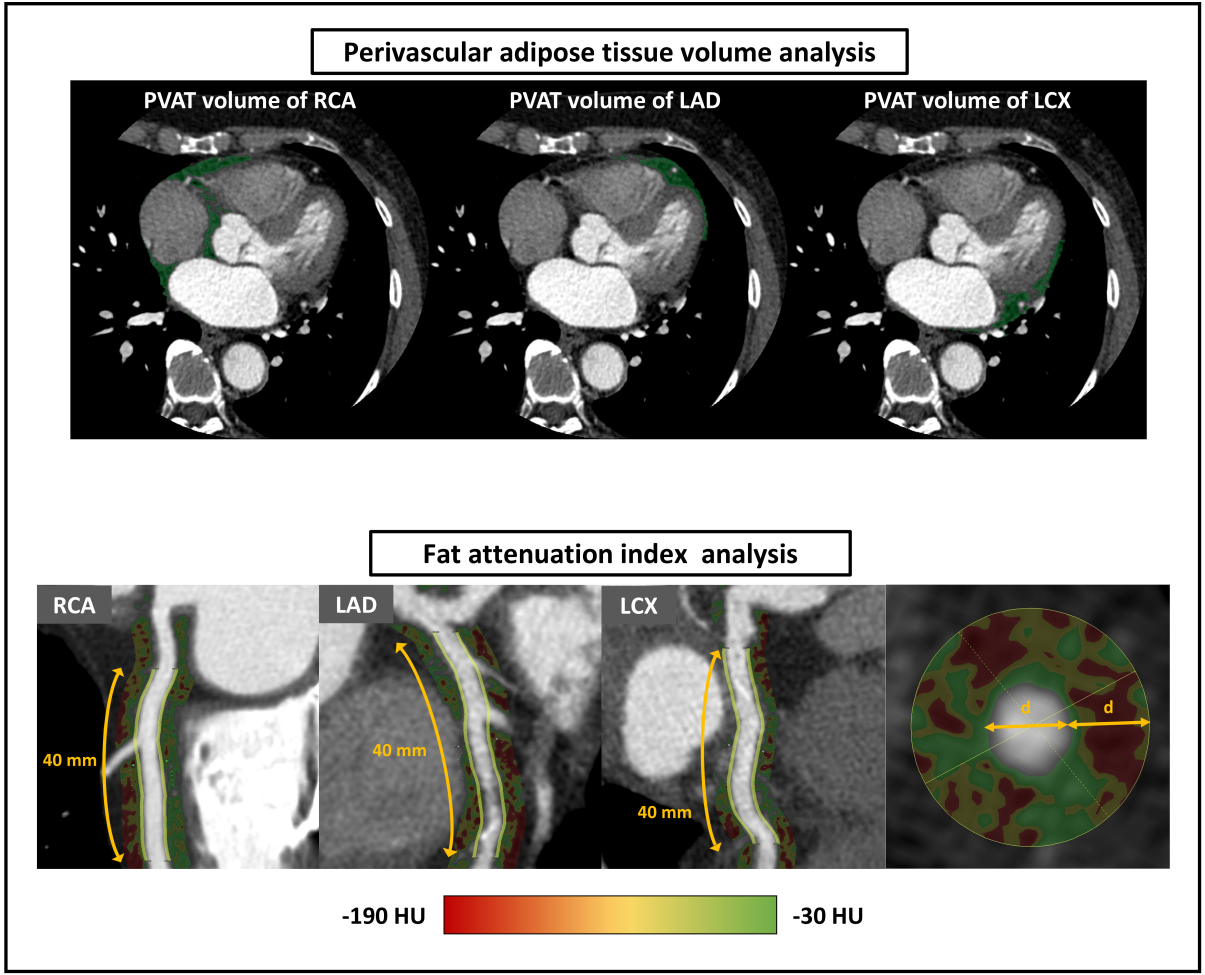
Coronary computed tomography angiography (CTA) analysis for
